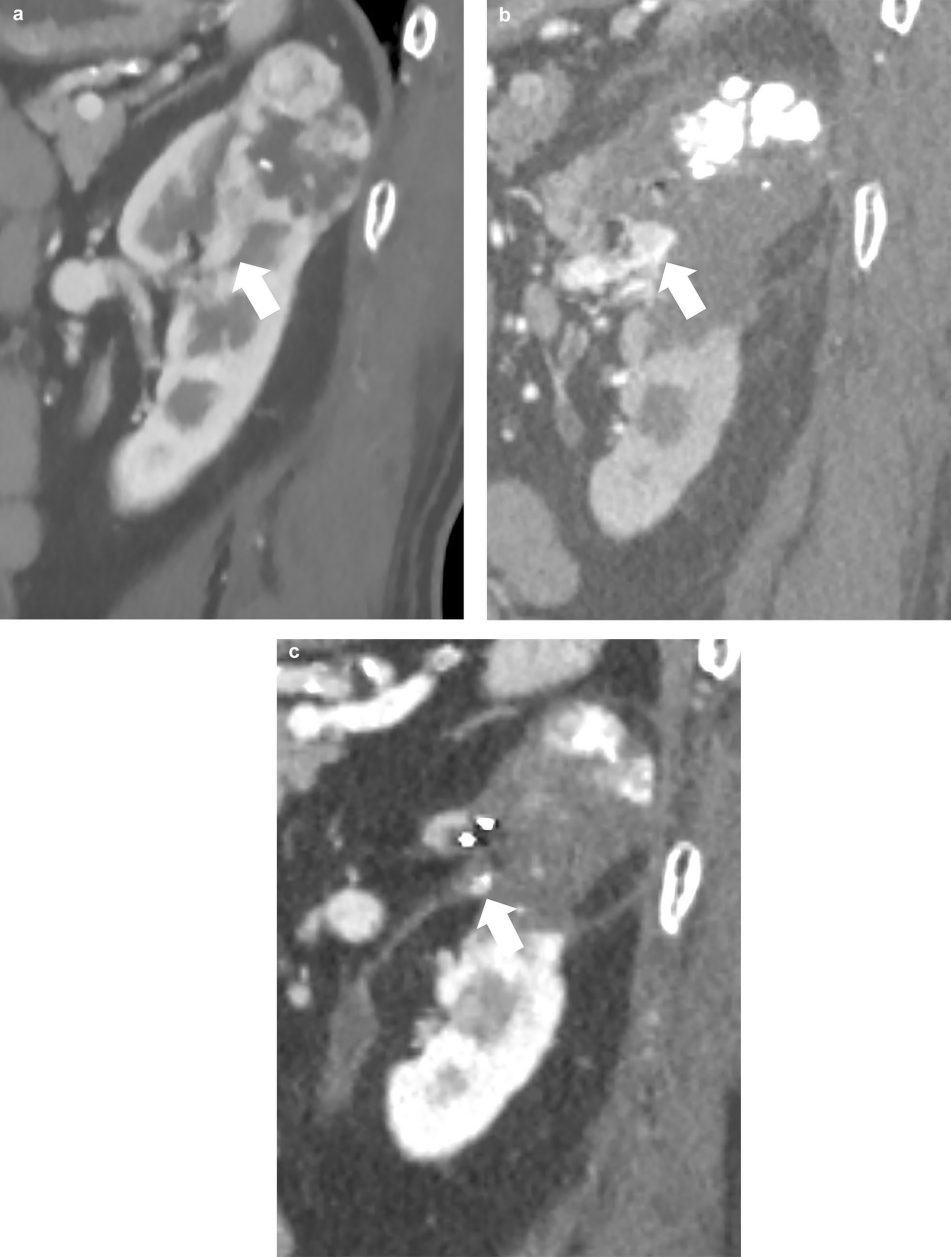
CT images of 4.4 cm T3a RCC in the left kidney in a 74 year-old man. a Sagittal CT image before treatment shows target RCC with the extension part into the segmental vein (arrow). b Sagittal dynamic CECT image (corticomedullary phase) 1 month after initial cryoablation shows the enhanced extension part into the segmental vein without lipiodol accumulation (arrow). Second cryoablation was performed 1.5 months after the initial cryoablation. c Sagittal CT image 51 months after initial cryoablation shows completely ablated T3a RCC with lipiodol accumulation (arrow) without enhancement. *CT* computed tomography; *CECT* contrast-enhanced CT; *RCC* renal cell carcinoma
